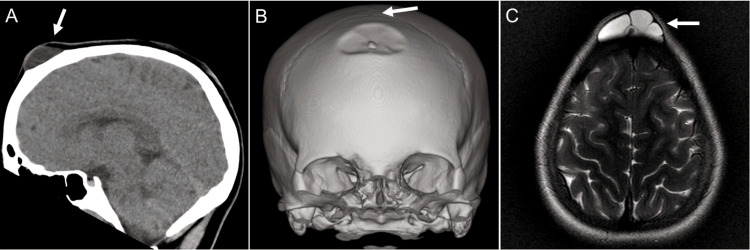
Radiographic examination of the lesion (A) A sagittal CT scan of the head demonstrates a soft tissue lesion (arrow) overlying the frontal bone; (B) Bone window reconstructions demonstrate indentation of the frontal bone which remains intact. The lesion is distant from bregma (arrow), the site of the now-closed anterior fontanelle; (C) T2-weighted MRI demonstrates the lobulated, T2-hyperintense lesion (arrow), distant from the brain
